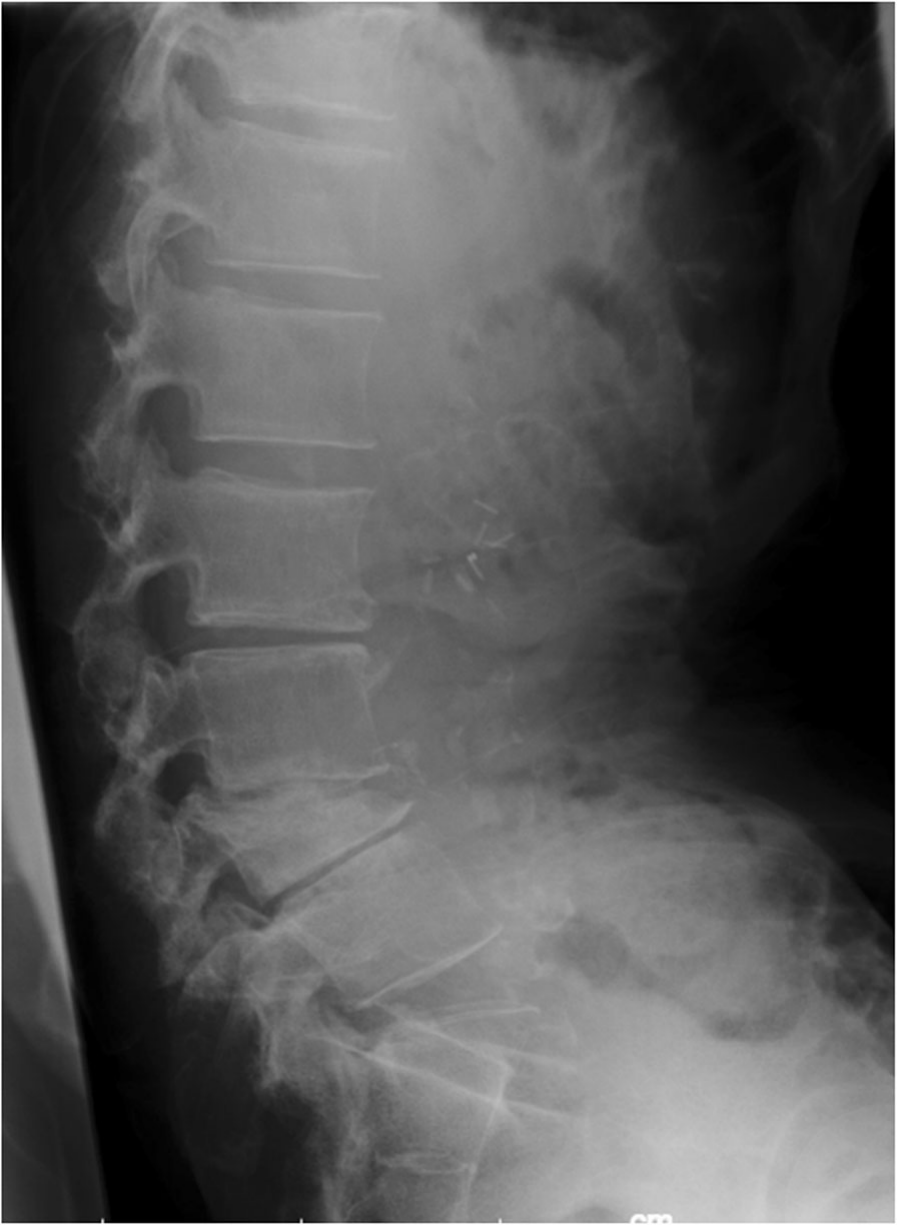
A radiograph of lateral lumbar spine. Compression fracture of L4 vertebral body is observed
